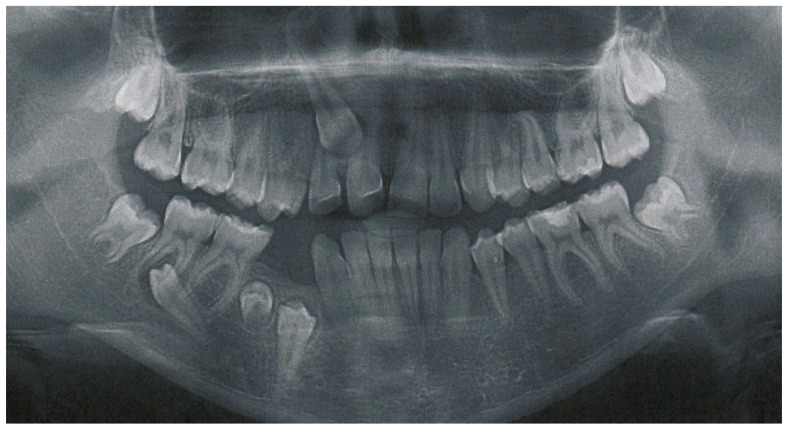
Panoramic radiograph, taken two months later, showing the continued distal migration of the tooth 45, here located between the roots of the tooth 47.
